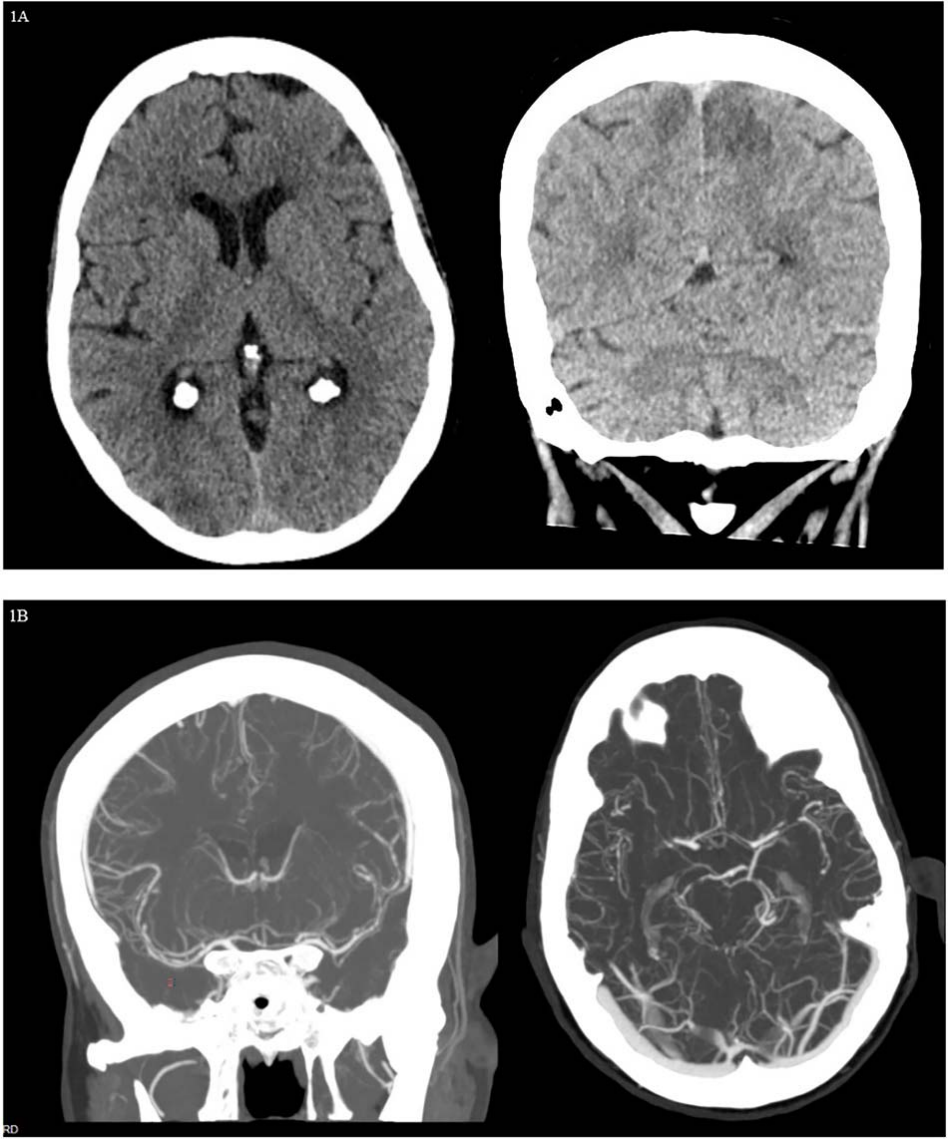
Case 1. CT head and CTA head and neck findings during the first admission. (A) CT head showing bilateral hypoattenuation within the paramedian parietal and occipital lobes. (B) CTA head and neck showing multifocal stenosis in bilateral MCAs and PCAs, along with bilateral multifocal luminal irregularities in ACAs.
